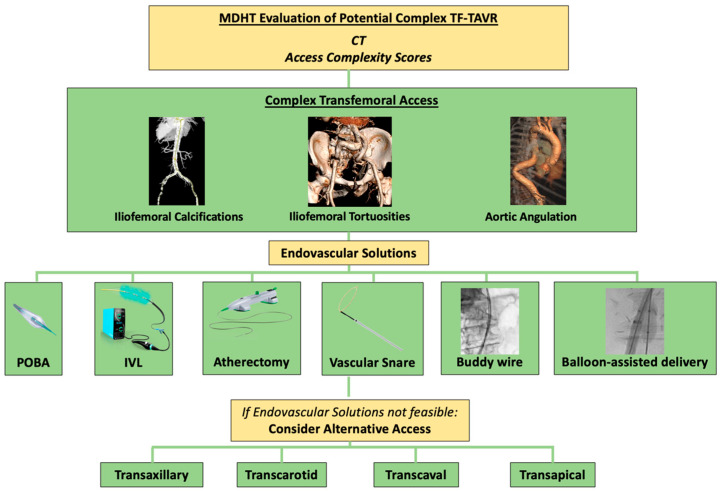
Proposed algorithm for potential challenges and solutions during complex TF-TAVR. CT, computed tomography; IVL, intravascular lithotripsy; POBA, plain balloon angioplasty; TF-TAVR, transfemoral access transcatheter aortic valve replacement.
